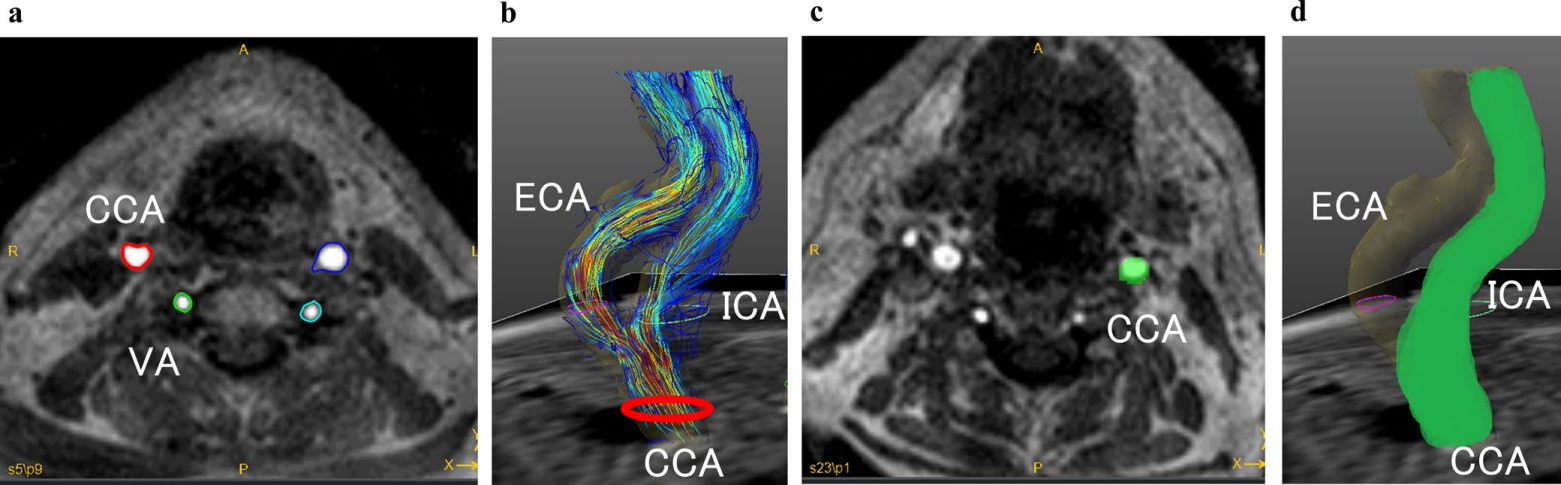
4D flow MRI magnitude images (a, c) and 3D images (b, d). BFV was measured by applying the semi‐automatic iso‐contour ROI in the proximal CCA (a, b). TKE was measured by manually defining the VOI from the CCA to the ICA (c, d). BFV = blood flow volume, CCA = common carotid artery, ICA = internal carotid artery, ROI = region of interest, TKE = turbulent kinetic energy, VOI = volume of interest.
